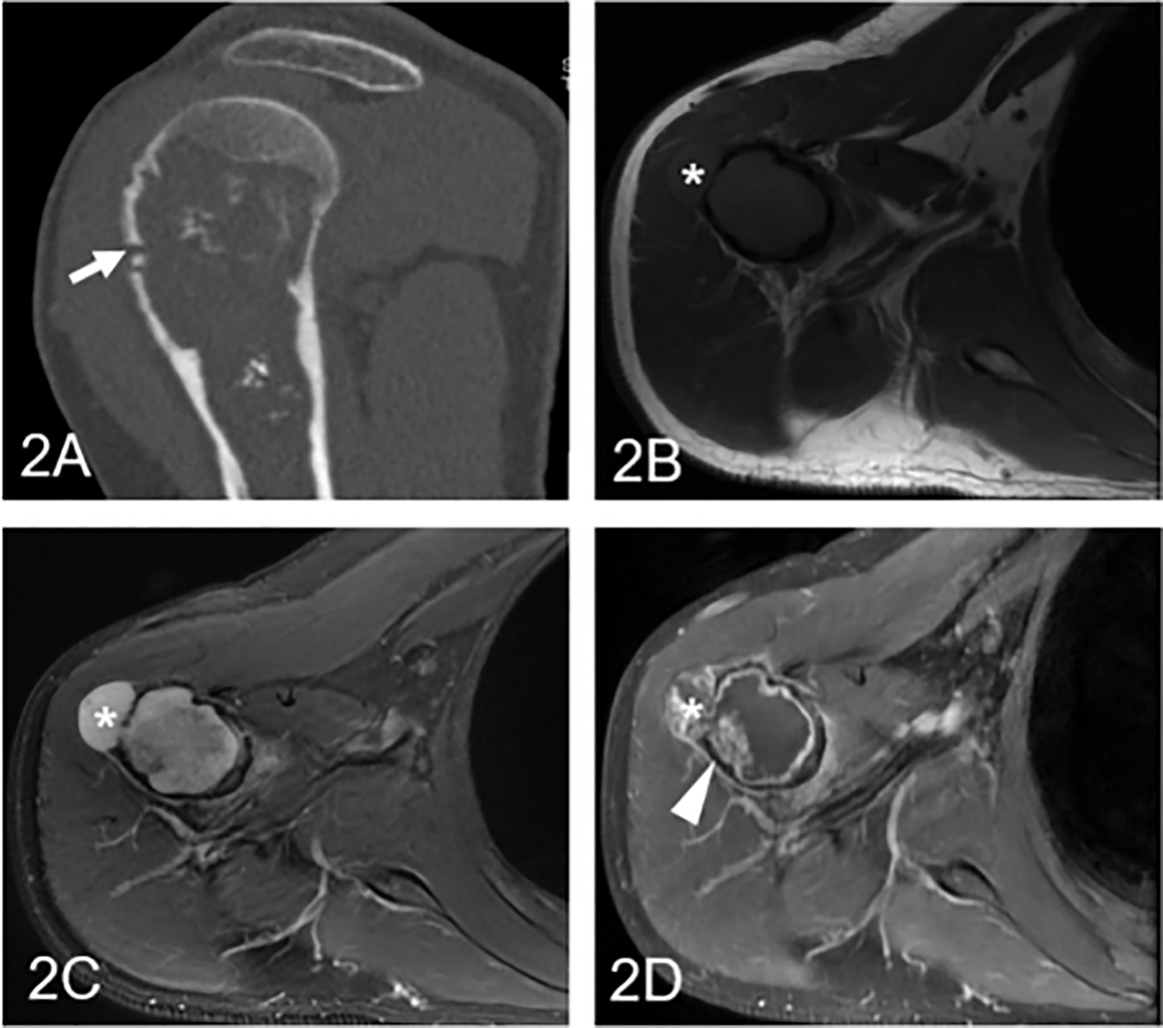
Low-grade chondrosarcoma involving the humerus in a 57-year-old man. (A) Coronal CT shows an ill-defined intramedullary osteolytic lesion with several ring and popcorn calcified components, and the arrowhead shows cortical disruption (arrow). (B) Axial T1WI presents a homogeneous lesion with an associated soft tissue mass (asterisk). (C) Axial fat-suppressed T2WI demonstrates a heterogeneous hyperintense lesion with a soft tissue component (asterisk) and endosteal scalloping of the cortex. (D) Coronal fat-suppressed contrast-enhanced T1WI demonstrates patched (arrowhead) and marginal enhancement.
